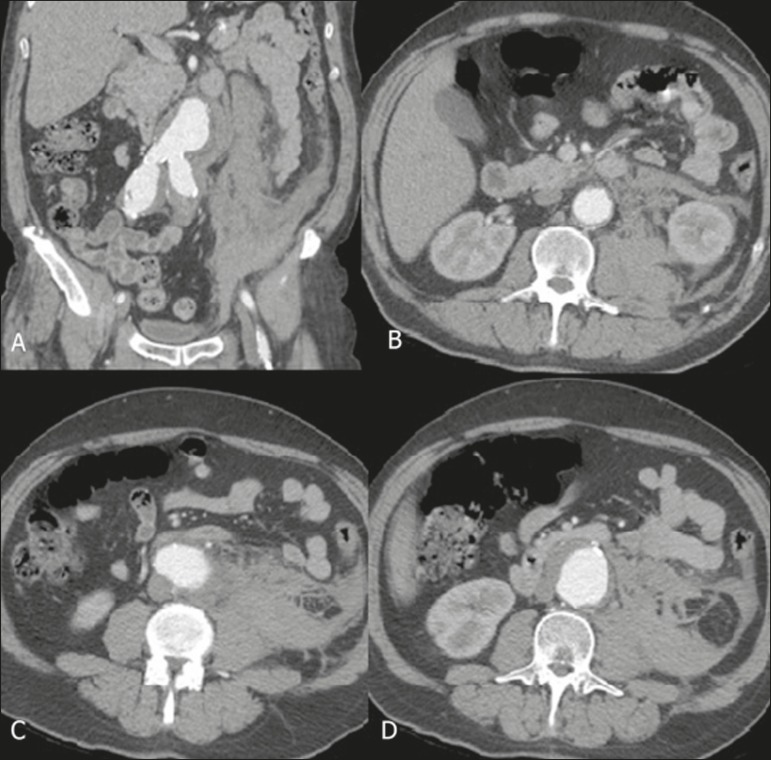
CT angiography with coronal reconstruction (A) and axial
